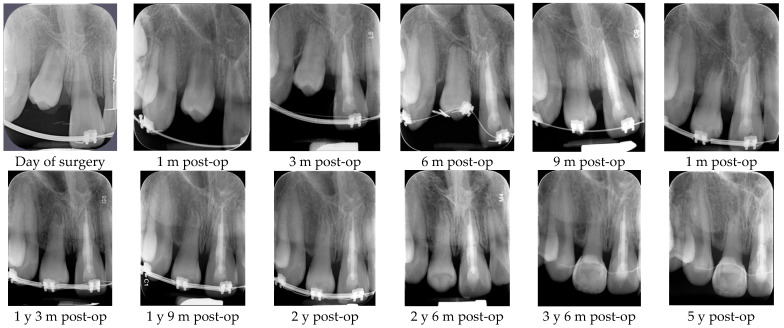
Sequential periapical radiographs of tooth 44 in the five years following the autotransplantation. Time elapsed in years (y) and months (m). Dimensions: 3.05 × 4 cm.
